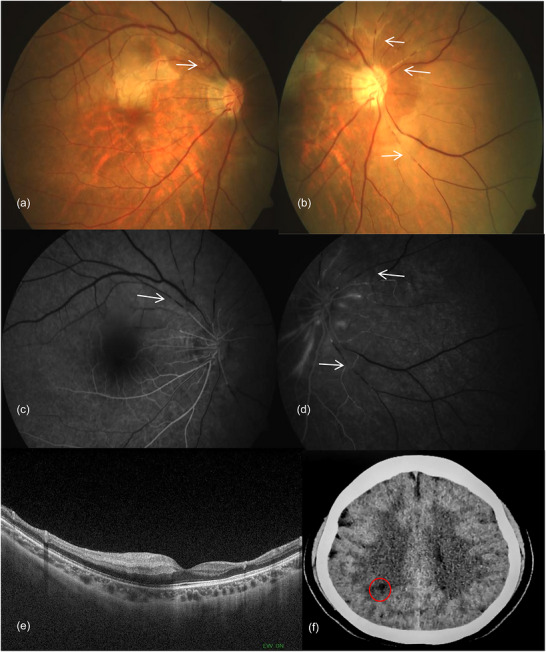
(a, b) Fundus photography shows thinning of the retinal artery; white arrows indicate segmental occlusion of the retinal artery in the right eye(OD). The nasal and superior retina appear grey–white and oedematous, and the retina above the macula is also grey–white and oedematous. (c, d) Fluorescein angiography demonstrates a filling defect in the arterial phase in the superior hemisphere and nasal region, with white arrows indicating non‐perfused vessels. (e) Optical coherence tomography shows retinal oedema and thickening above the fovea. (f) Non‐contrast cranial CT demonstrates a lacunar infarct in the right parietal lobe, with a circle marking the location of the infarction.
